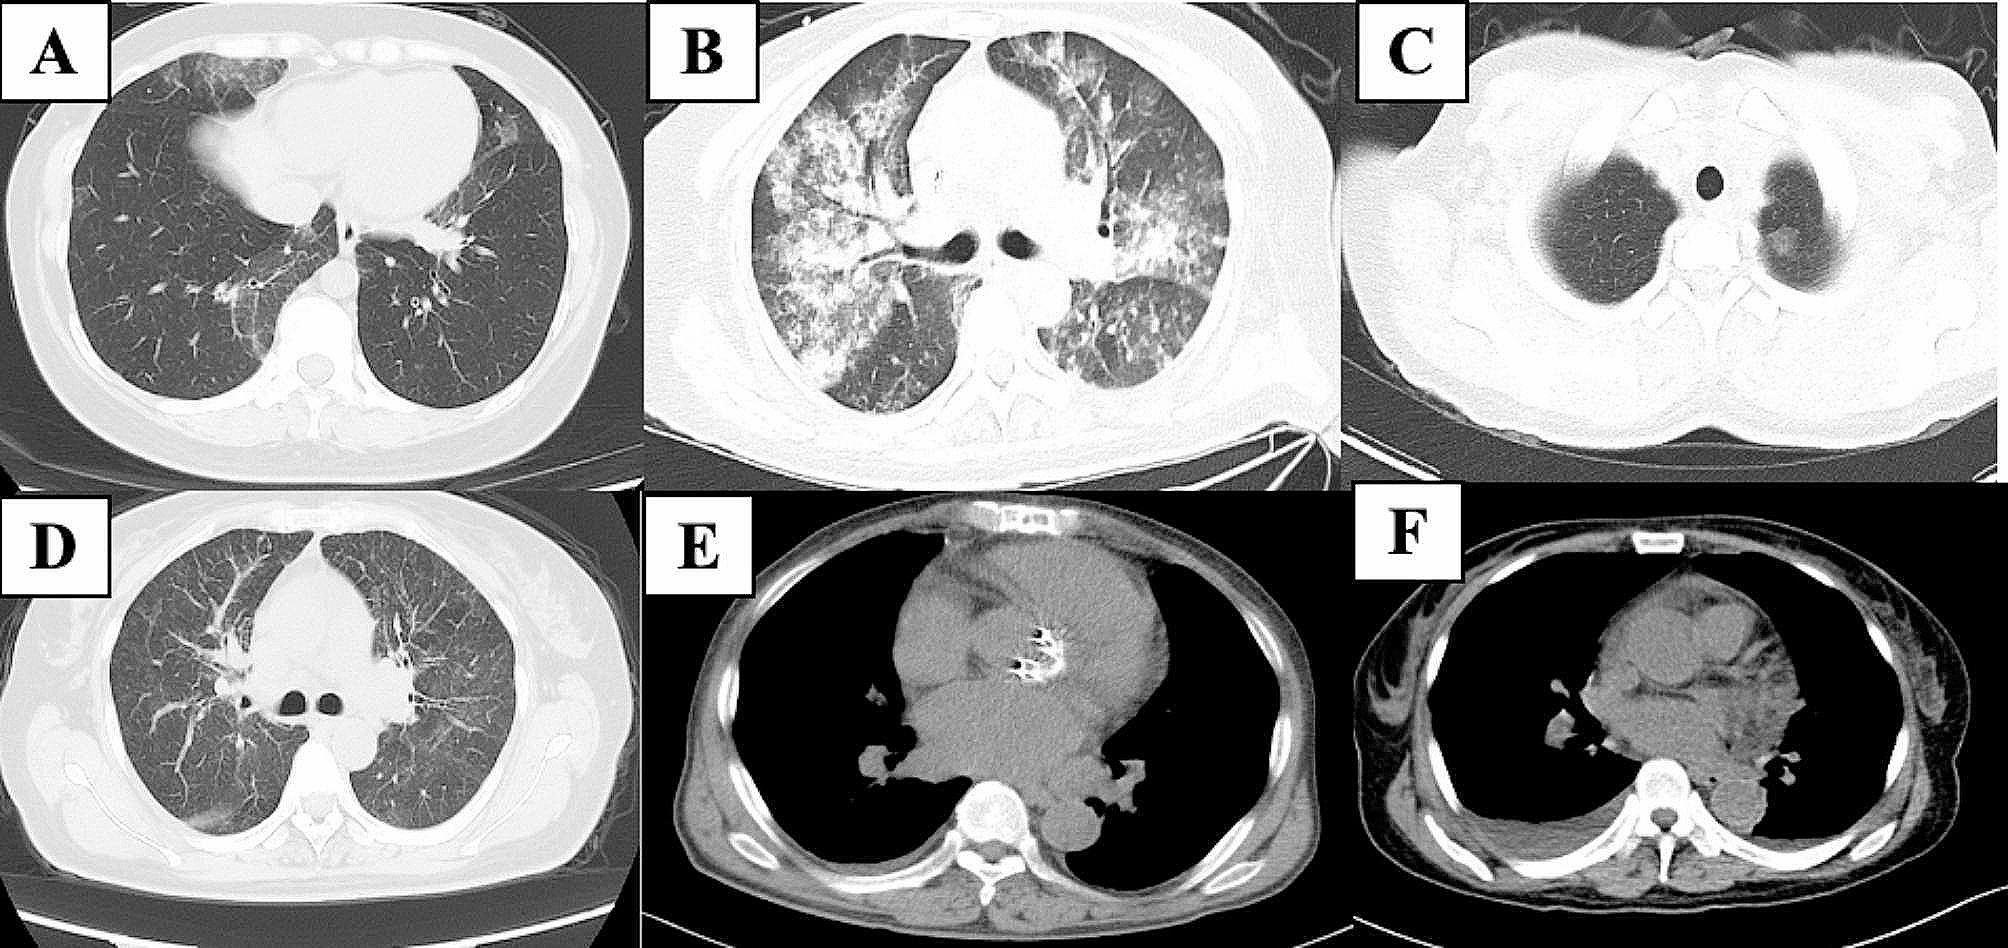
A-E, CT imaging findings associated with cryoglobulinemia and lung involvement. A, Diffuse, bilateral, patchy GGOs. B, Diffuse alveolar hemorrhage. C, Solitary large nodule in the right upper lobe. D, Diffuse, bilateral, multiple, small nodules. E, Bilateral pleural thickening. F, Bilateral pleural effusions
